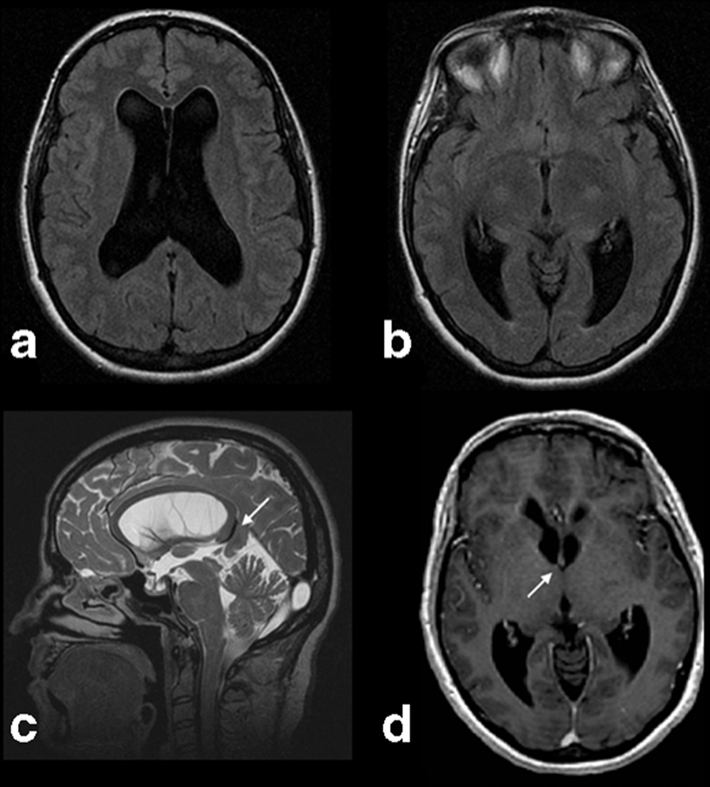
(a, b) Axial FSE *T*2 FLAIR WI of the whole brain shows normal periventricular signal intensity of the white matter and a slit-like third ventricle. (c) Sagital 3D CUBE *T*2WI confirmes the absence of dilatation or morphologic alterations of the third ventricle, along with a normal Silvio aqueduct. A subtle precuneus herniation due to focal tentorial hypoplasia was also noticed (white arrow). (d) Axial FSPGR 3D *T*1WI after i.v. contrast agent administration showed no abnormal enhancements. A stenotic foramina of Monro were found, mainly on the right side (white arrow). 3D, three-dimensional; FLAIR, fluid attenuated inversion recovery; FSE, fast spinecho; FSPGR, fast spoiled gradient echo; *T*1WI, *T1* weighted imaging; *T*2WI, *T2* weighted imaging.
